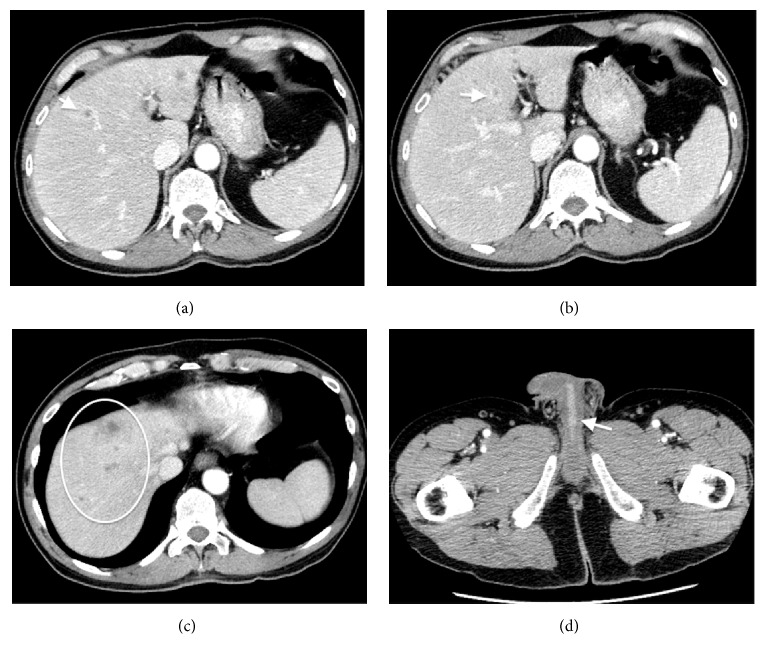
Four axial contrast-enhanced CT images of the abdomen and pelvis. ((a) and (b)) Several rim enhancing lesions are identified in the right and left hepatic lobes (arrows). (c) Nonenhancing hepatic lesions at the hepatic dome (circle); (d) axial image through the inferior pelvis with prominent enhancement along the urethra (arrow).
